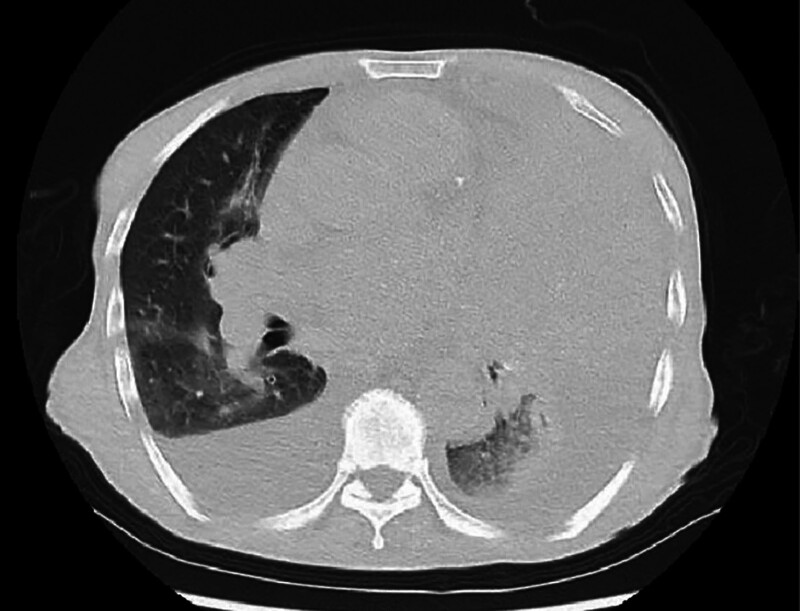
Follow-up chest CT demonstrating progression of left upper lobe mass with bilateral pleural effusions. CT = computed tomography.
